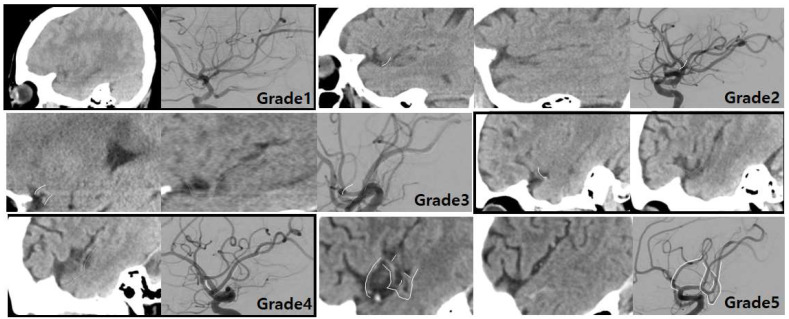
NCT grading of distal-to-thrombus segment in correspondence to the reference standard of final DSA findings. NCT showed no MCA course at all (grade 1), only a small portion of the inferior division of MCA (grade 2), almost all portions of the inferior division of MCA (grade 3), a small portion of the inferior division and almost all of the superior division of MCA (grade 4), and all of the inferior division and superior division of MCA (grade 5), respectively. The arterial courses on NCT were matched with those on DSA as indicated by solid and dotted lines. NCT, non-contrast computed tomography; DSA, digital subtraction angiography; MCA, middle cerebral artery.
